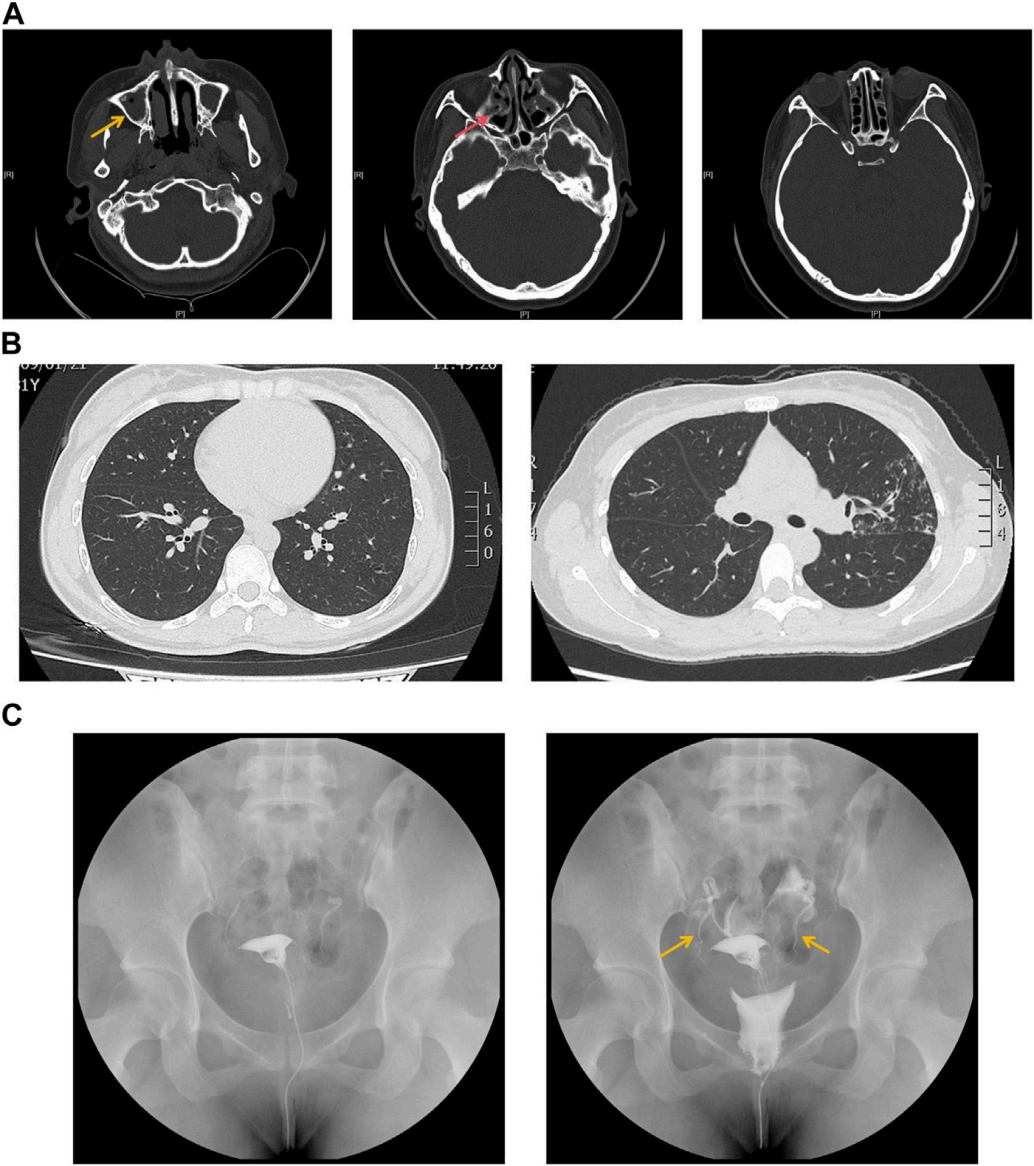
(A) Sinus computed tomography showed inflammation in both maxillary (yellow arrow) and ethmoidal sinuses (red arrow). (B) Chest computed tomography showed bronchiectasis in both sides of lung lobes. (C) Salpingography showed no obstruction in bilateral fallopian tubes but was not patent like as normal (yellow arrow).
